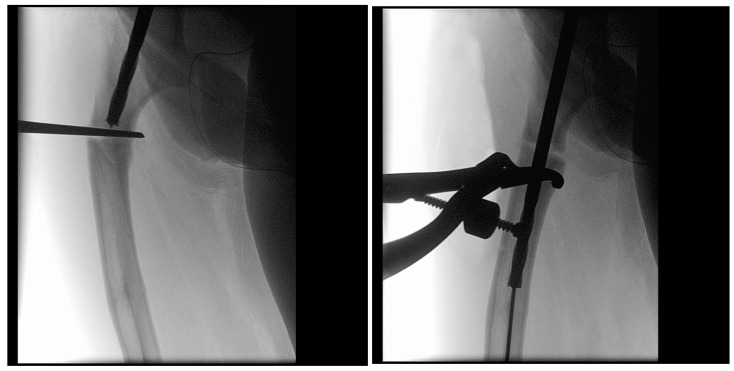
Intraoperative X-rays showing the first CORA and alignment with the continuation of the reaming.
